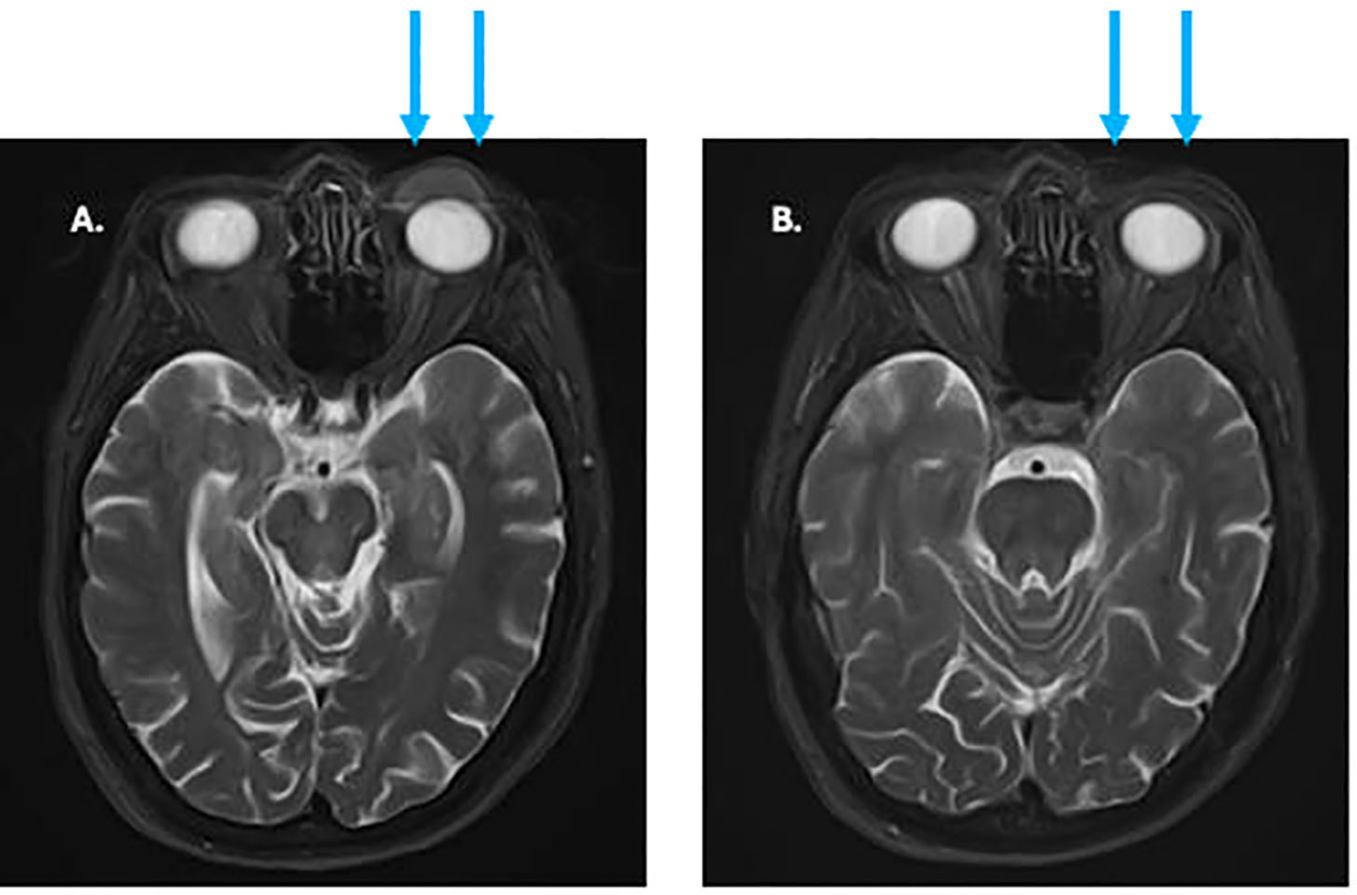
(A) pre-treatment MRI of facial soft tissues showing eyelid tumor (arrows). (B) MRI after 3 months of treatment showing complete regression of previous tumor (arrows).
